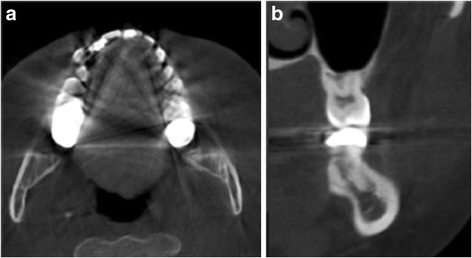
Postoperative computed tomography imaging shows that the calcified masses were excised completely. a Axial. b Coronal
